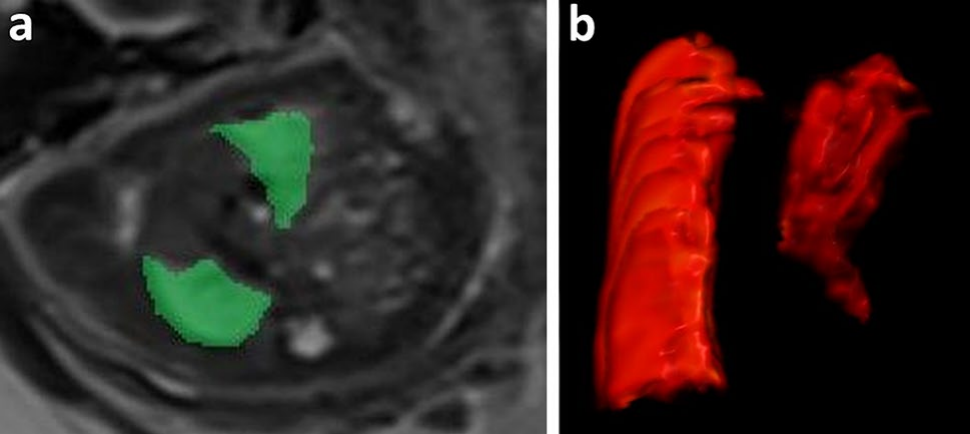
Total fetal lung volume (TFLV) measurement. a T2 HASTE axial image showing the lung segmentation methods tracing the region of interest (green) around the left and right lung on each MRI slice. b 3D volume rendering reconstruction of the TFLV, obtained by summing each slice area, multiplied by the slice thickness
